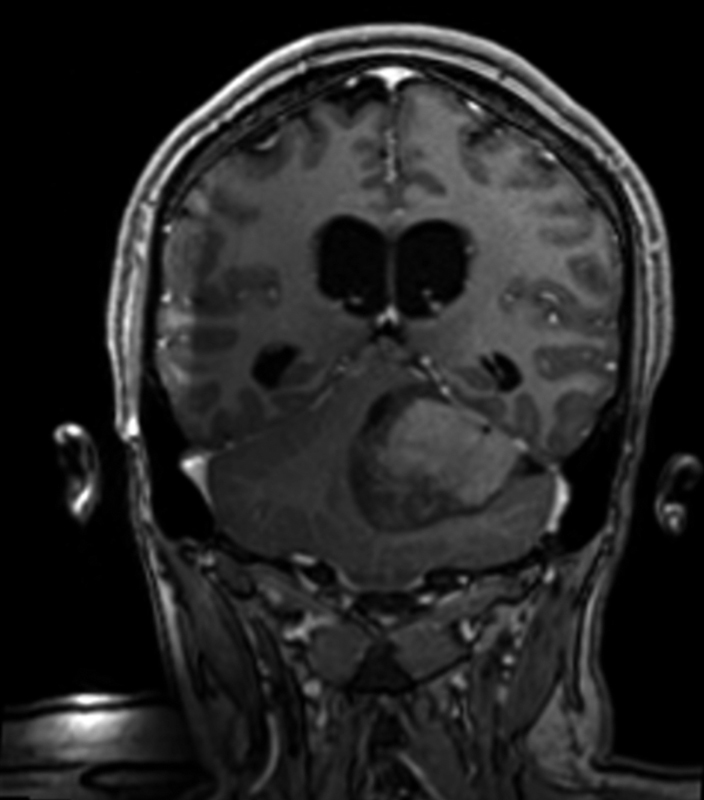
Magnetic resonance imaging of the skull shows expansive lesion in the posterior fossa with epicenter in the cerebellar vermis/IV ventricle, solid and cystic.
